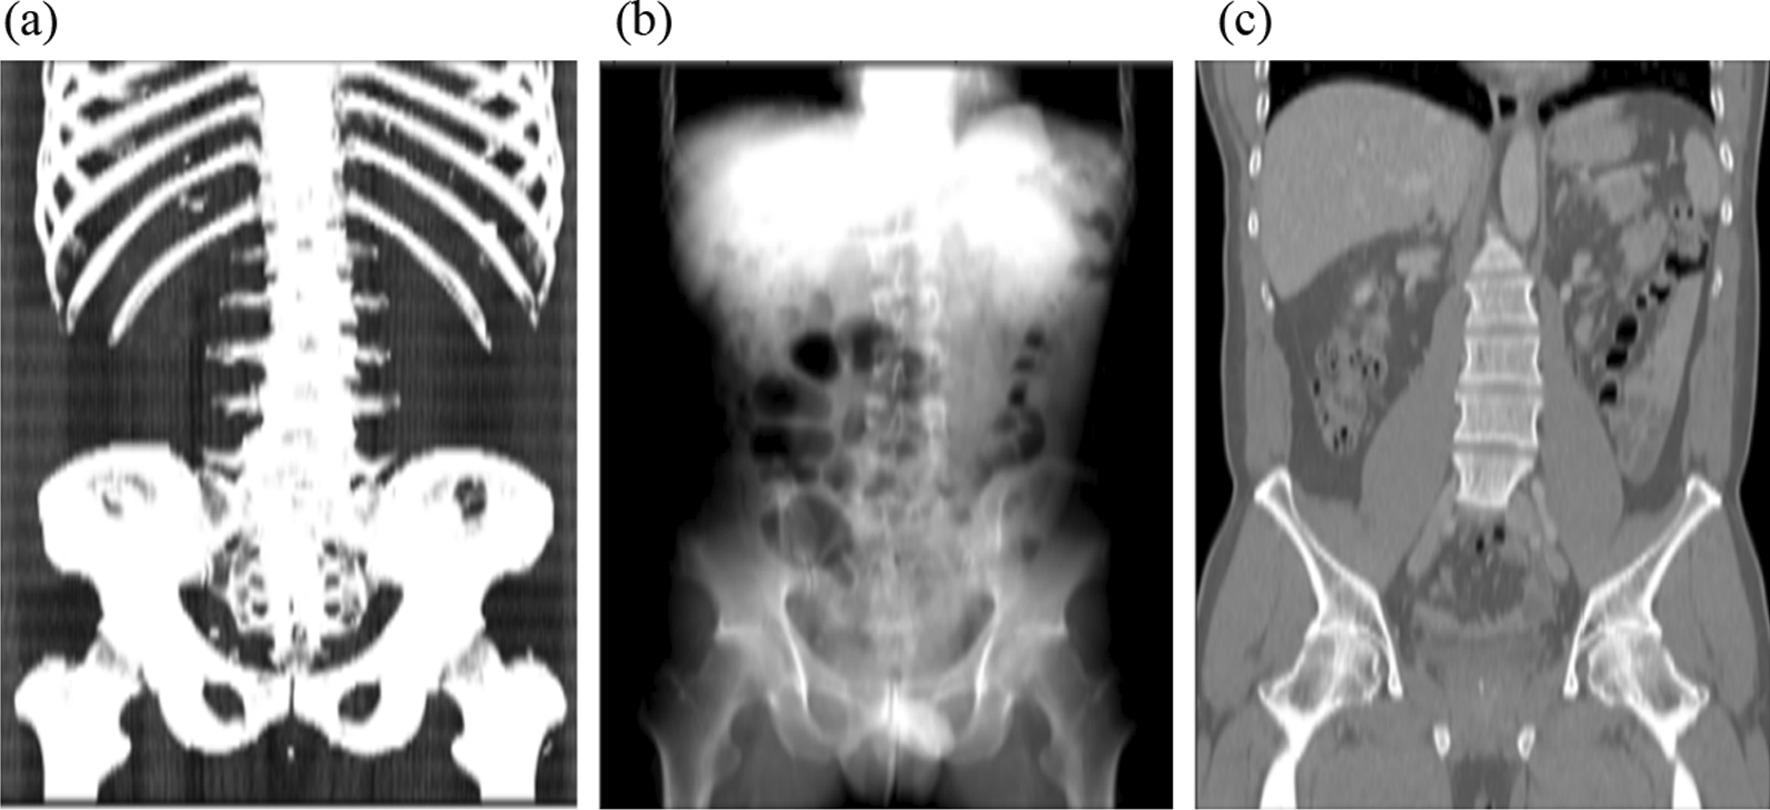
The image reconstruction method used in this study. The 3D CT image data with multiple slices are transformed into a 2D representative images using the maximum intensity projection in a coronal image (MIP_coronal_) (a), average intensity projection (AIP_coronal_) (b), and mid-coronal plane (c). In this example, we used a contrast-enhanced abdomen and pelvis CT
